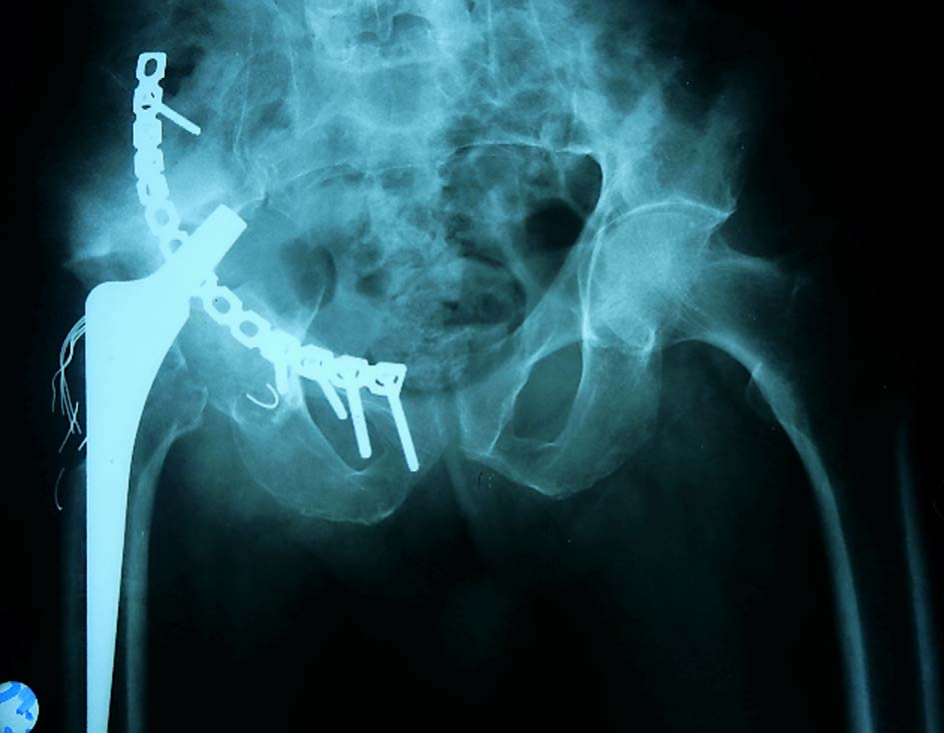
Intra-operative X-ray, the cup liner construct and the femoral head had been removed. Plating of the anterior column was done.
